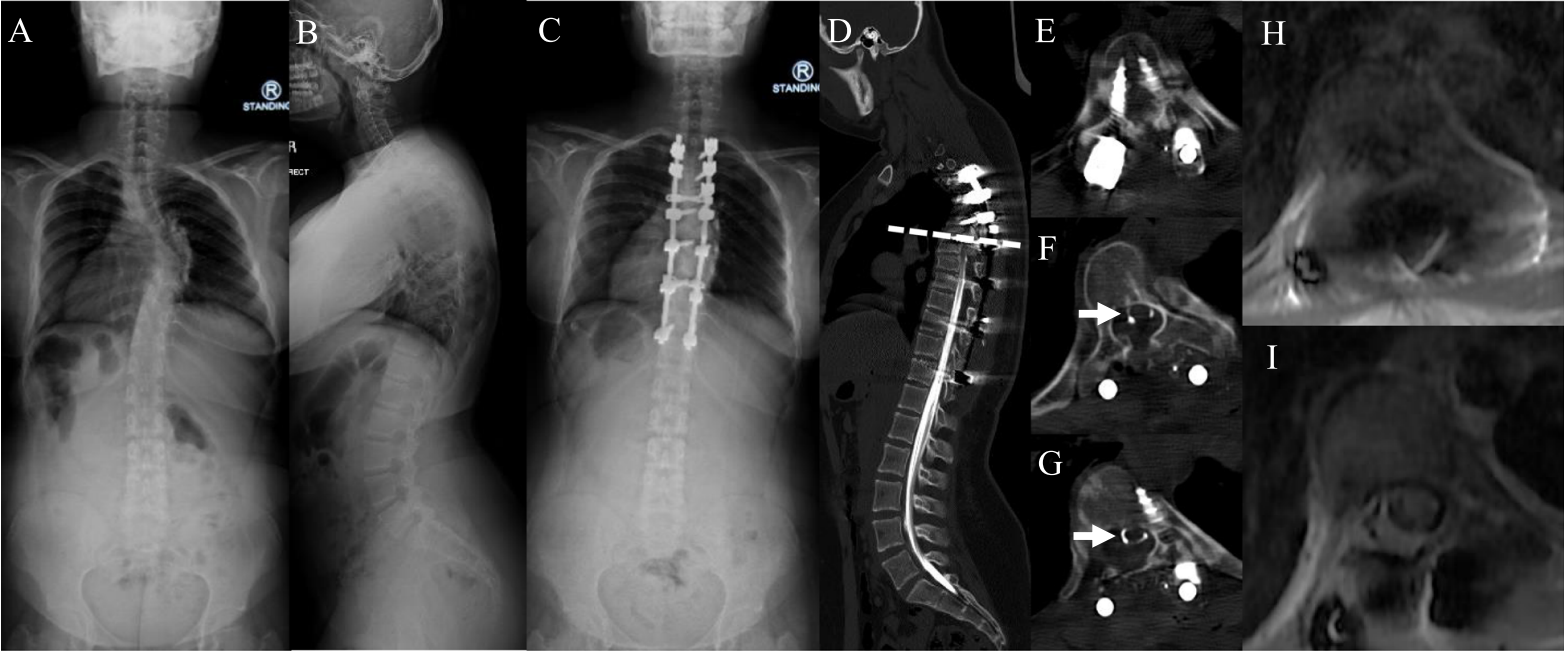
One patient (preoperative x-ray – a & b, postoperative AP x-ray – c) developed transient neurological deficit upon reversal of anesthesia. Urgent computed tomography (CT) myelography demonstrated cessation of contrast at T5 pedicle level in the sagittal view (dashed line in d) and axial view (e). Axial view at 5 mm (f) and 10 mm (g) below T5 level showed a thin layer of contrast within the thecal sac. Magnetic resonance imaging (MRI) showed epidural hematoma with spinal cord compression at T5/6 level (h). The spinal cord was visible at 15 mm below T5/6 level (i). Clinical assessment, MRI whole spine and CT thoracolumbosacral did not show any secondary causes for scoliosis
